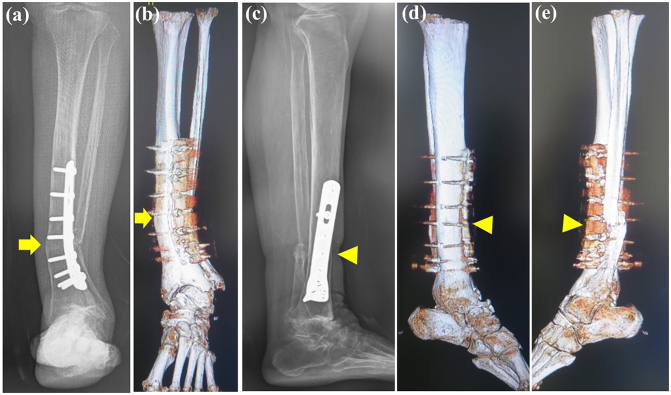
Valgus malalignment of the tibia as revealed by (a) a standing anteroposterior radiograph and (b) a 3-dimensional computed tomography scan (arrow). Recurvatum malalignment deformity of the tibia as revealed by (c) a standing lateral radiograph and (d) medial and (e) lateral 3-dimensional computed tomography scans (arrowhead).
